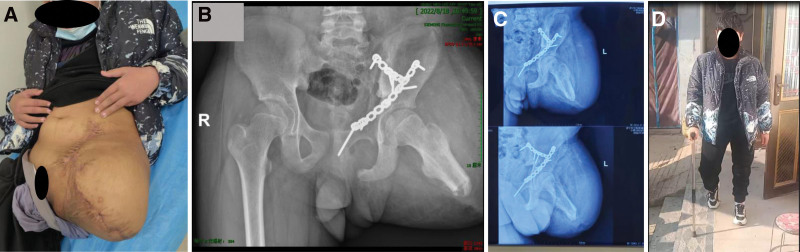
(A) The left lower abdominal and hip wounds were well healed at discharge. (B) X-rays at discharge showed a well-matched sacroiliac joint. (C) X-rays at 7 months postoperatively showed that the fracture had healed. (D) The patient was fitted with a prosthesis 1 year later and was doing functional exercises for walking.
